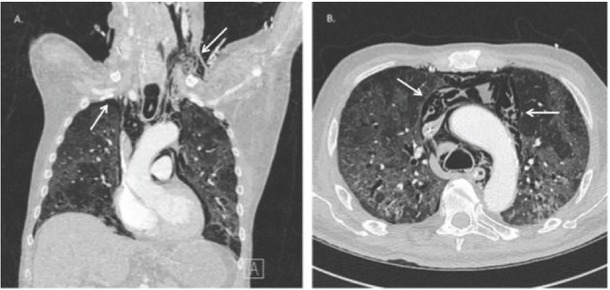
Chest CT showing (A) a right-sided apical pneumothorax and subcutaneous emphysema up to the left side of the neck and (B) extensive pneumomediastinum * Paw: airway pressure (cmH_2_O), Pes: esophageal pressure (cmH_2_O), PL: transpulmonary pressure (cmH_2_O)
